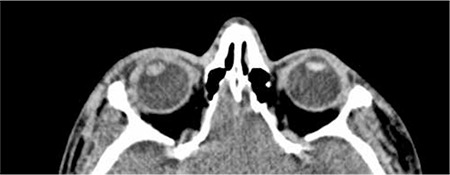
Preoperative orbital computed tomography image
